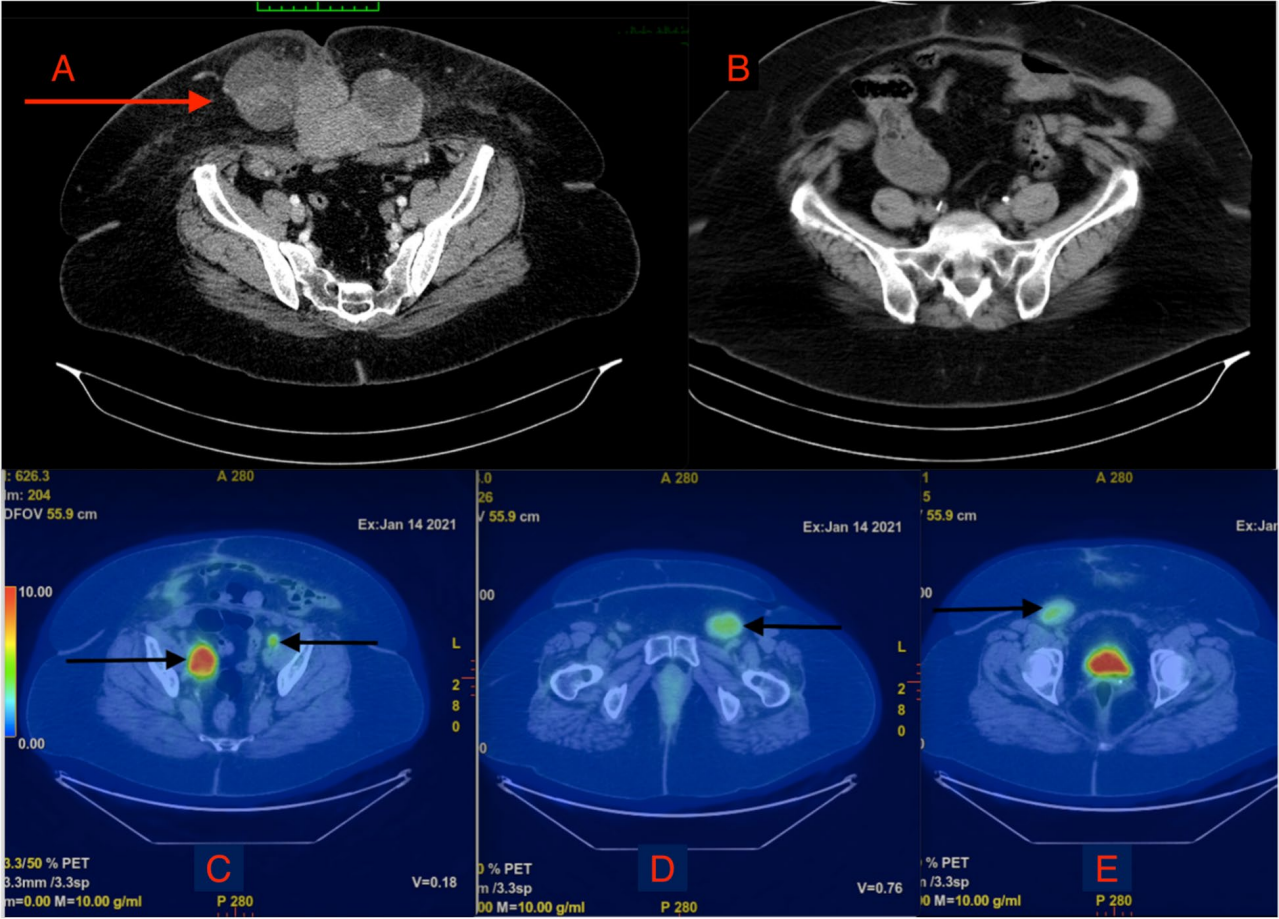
Axial CT images of the abdomen and pelvis. A Before resection and B after surgery—laparotomy with the removal of the abdominal wall mass, lymph nodes, and closure with polypropylene mesh. PET scan images (C, D, E) demonstrating recurrent carcinoma in the pelvic, left inguinofemoral, and right inguinofemoral nodes, respectively
